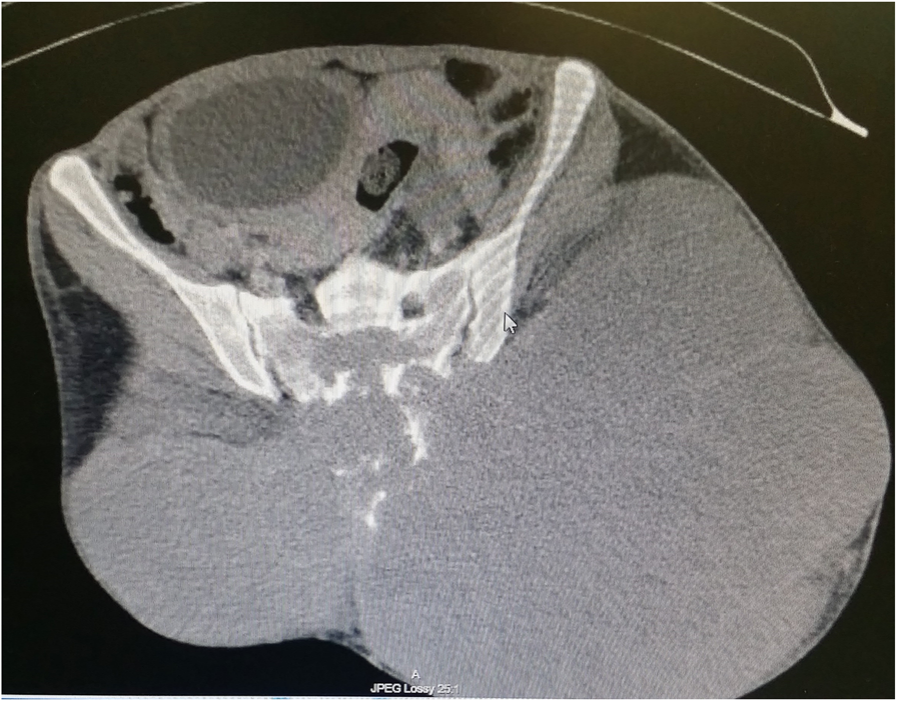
Coronal view Computed Tomography Scan of Pelvis
